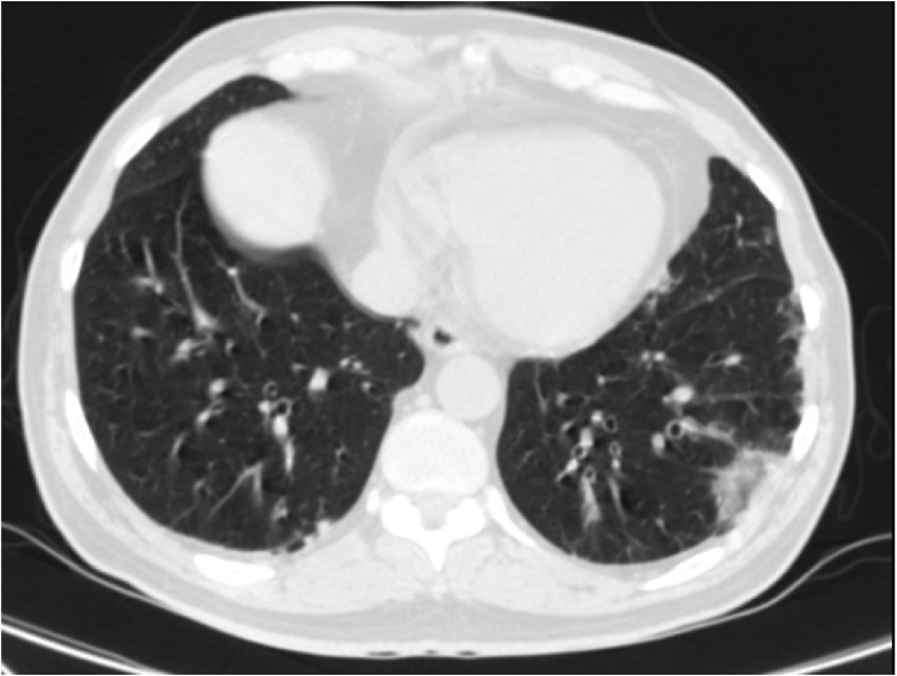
Influenza A viral infection: thin section CT shows subpleural GGO in left lower lobe
